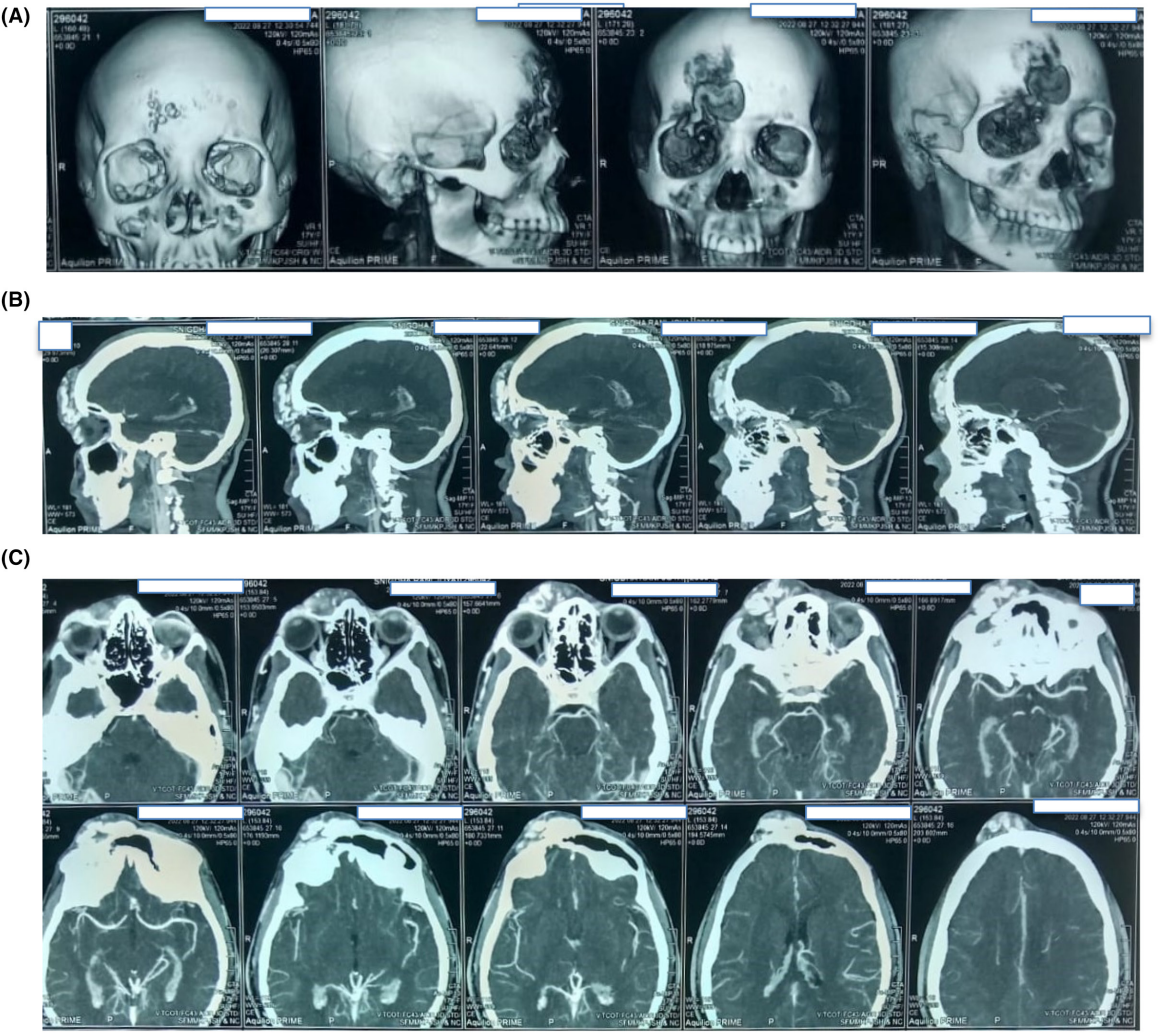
CT Scan of the brain with contrast showing right frontal extracalvarial arteriovenous malformation. (A) Superficial view showing bony defect, (B) Sagittal section, and (C) Transverse section.
